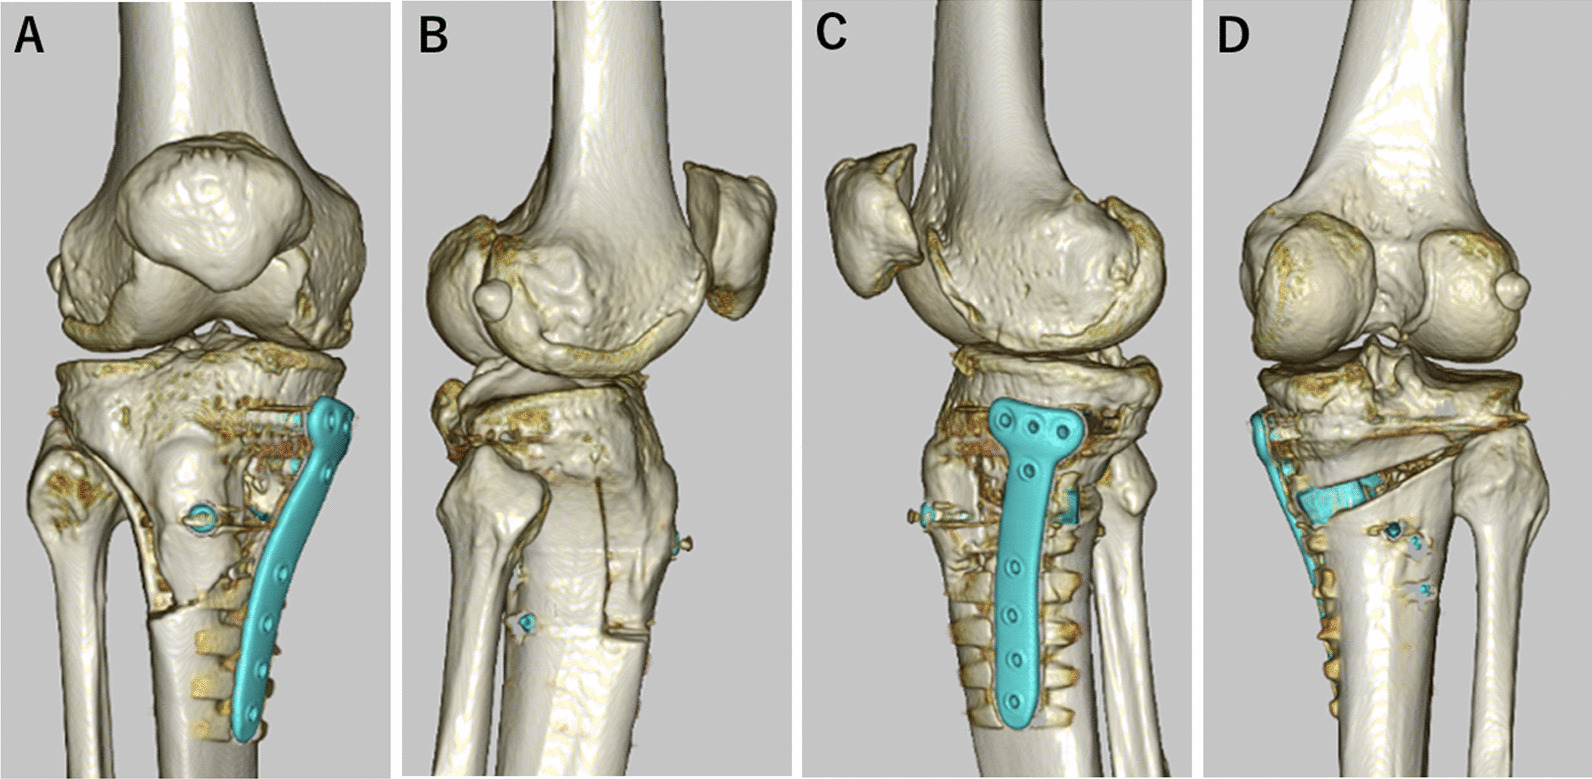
Postoperative three-dimensional computed tomography after medial opening-wedge distal tibial tuberosity osteotomy for right knee. A anteroposterior view. B lateral view. C medial view. D posteroanterior view
